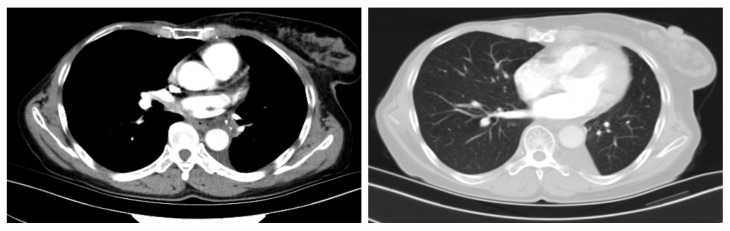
A follow-up CT scan revealed postoperative changes with no evidence of disease recurrence.
